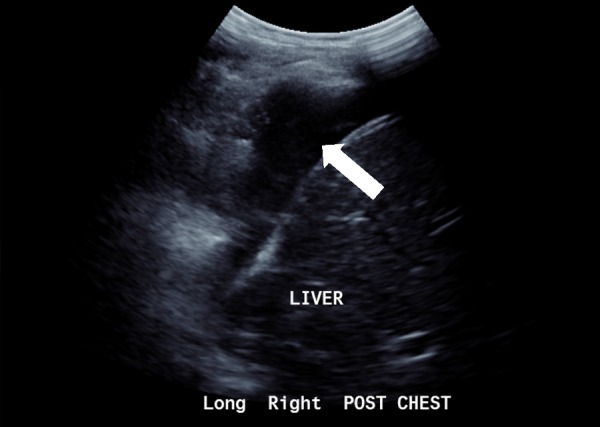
Ultrasound chest – complex septated right-sided effusion with associated low level internal echoes.
